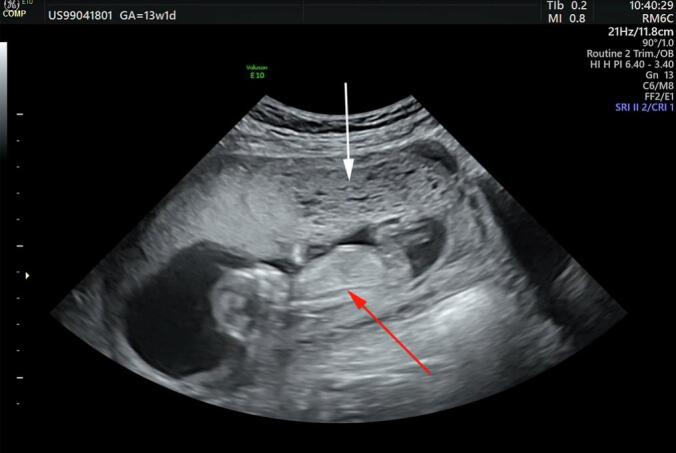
Ultrasound findings at 15 weeks of gestation demonstrating normal fetal parts (red arrow) alongside molar placenta (white arrow). (For interpretation of the references to colour in this figure legend, the reader is referred to the web version of this article.)
